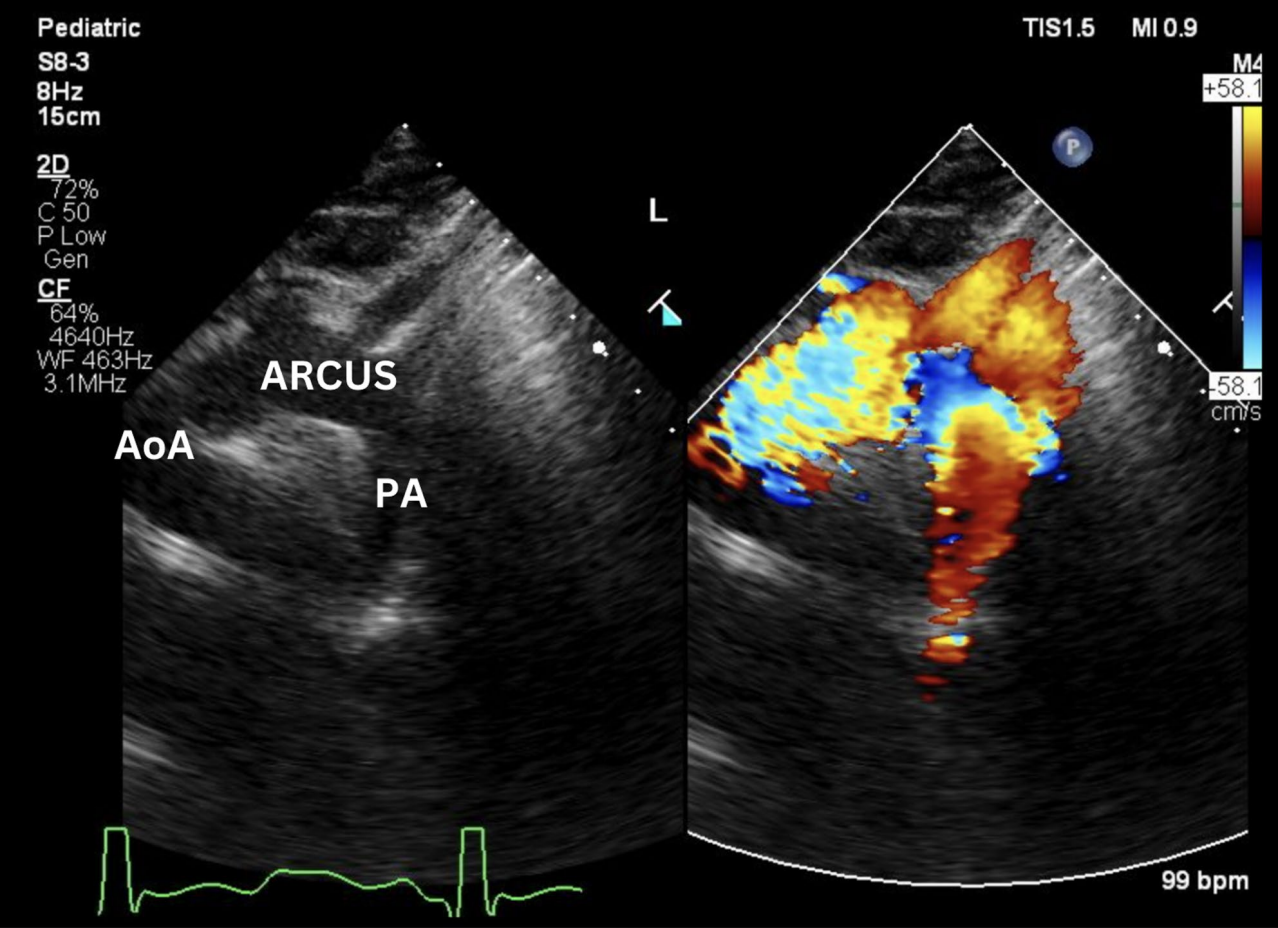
Post-operative suprasternal transthoracic echocardiogram showing complete closure of the aortopulmonary window (APW) between the ascending aorta (AoA) and main pulmonary artery (PA), with no residual flow detected on color Doppler
